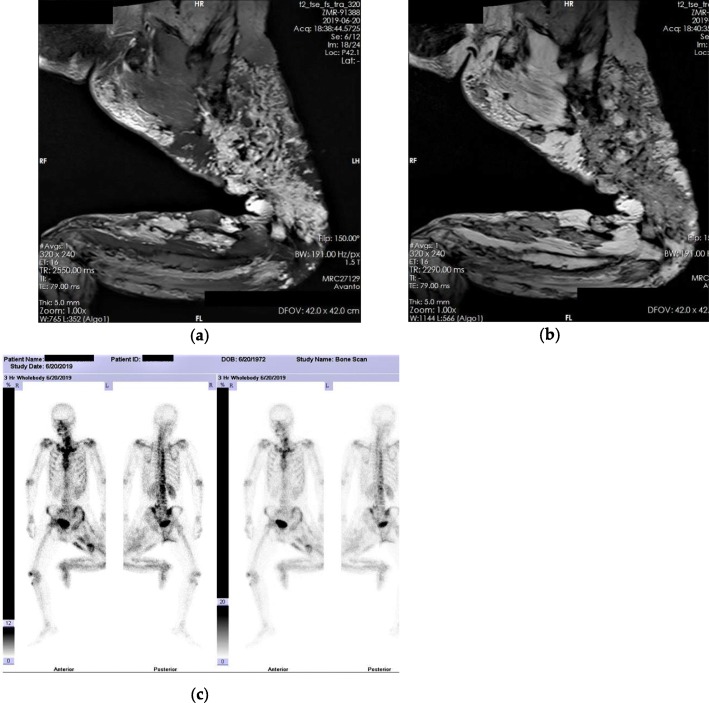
Additional imaging studies. a-b Magnetic Resonance Imaging (MRI) shows extensive high signal foci within the muscles; c Technetium 99 m-methyl diphosphonate (99mTc-MDP) bone scan shows radioactivity concentration at the flexor side of the limb
